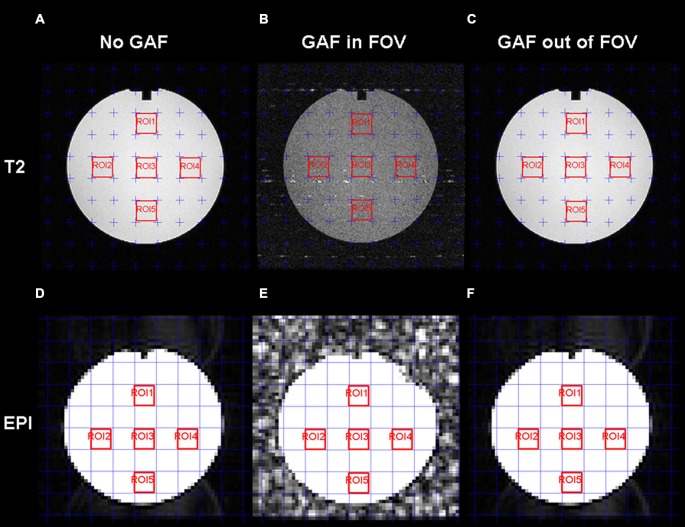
T2-weighted (A,B,C) and EPI (D,E,F) images with the five ROIs delineated in red for the SNR estimation. For each series, the three conditions are displayed: No GAF (A,D), GAF in FOV (B,E), and GAF out of FOV (C,F).
